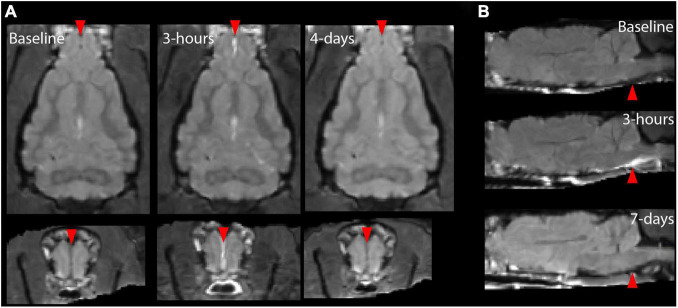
Individual T2 FLAIR post-contrast images showing regions of meningeal enhancement for two different ferrets 3 h after severe TBI. For the ferret shown in panel (A), contrast enhancement was localized to the anterior midline region and for the ferret shown in panel (B), contrast enhancement was found surrounding the brain stem. The red arrows indicate regions where extra cerebral enhancement was observable by eye for this modal on the day of injury but not 4–7 days after or at baseline.
